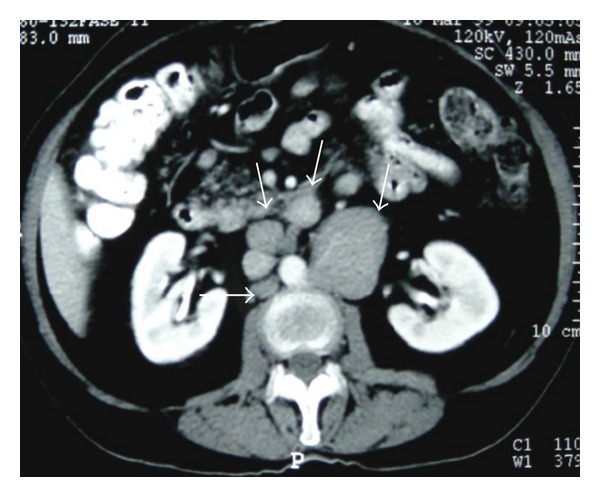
NHL. Axial contrast-enhanced abdominal CT shows retroperitoneal lymph nodes (arrows).
